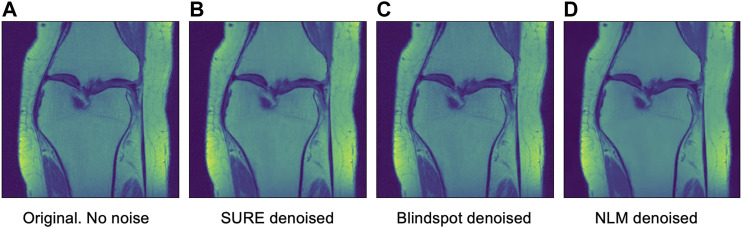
Example 2 of denoised brain MRI without adding any noise. The example image is the middle slice from one of the subjects. (A) Original image, no noise—(B) SURE denoised image—(C) Blindspot denoised image—(D) NLM denoised image.
